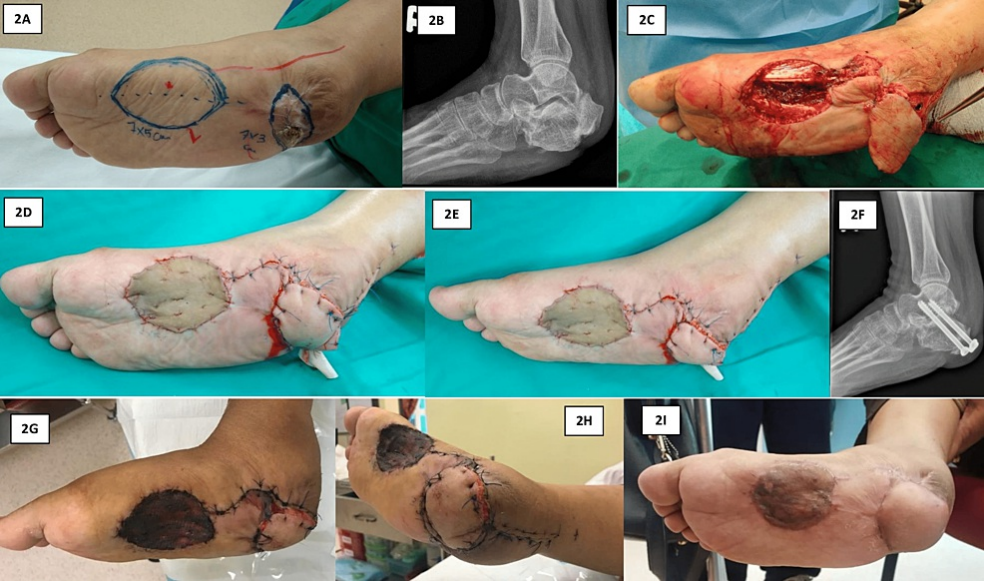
A: The patient presented with right foot equinus deformity and loss of heel pad with skin contracture. B: Plain radiograph of the ankle revealed a malunion of the calcaneum with incongruence of the subtalar joint. C: The medial plantar artery flap was raised intraoperatively. D and E: The medial plantar artery flap was anchored to the heel, and the donor site was covered with a full-thickness skin graft. F: Plain radiograph of the ankle revealed a successful subtalar fusion. G and H: The healing of the flap and skin graft was satisfactory six weeks after the surgery. I: The patient recovered fully with a near-normal range of movement of the ankle.
